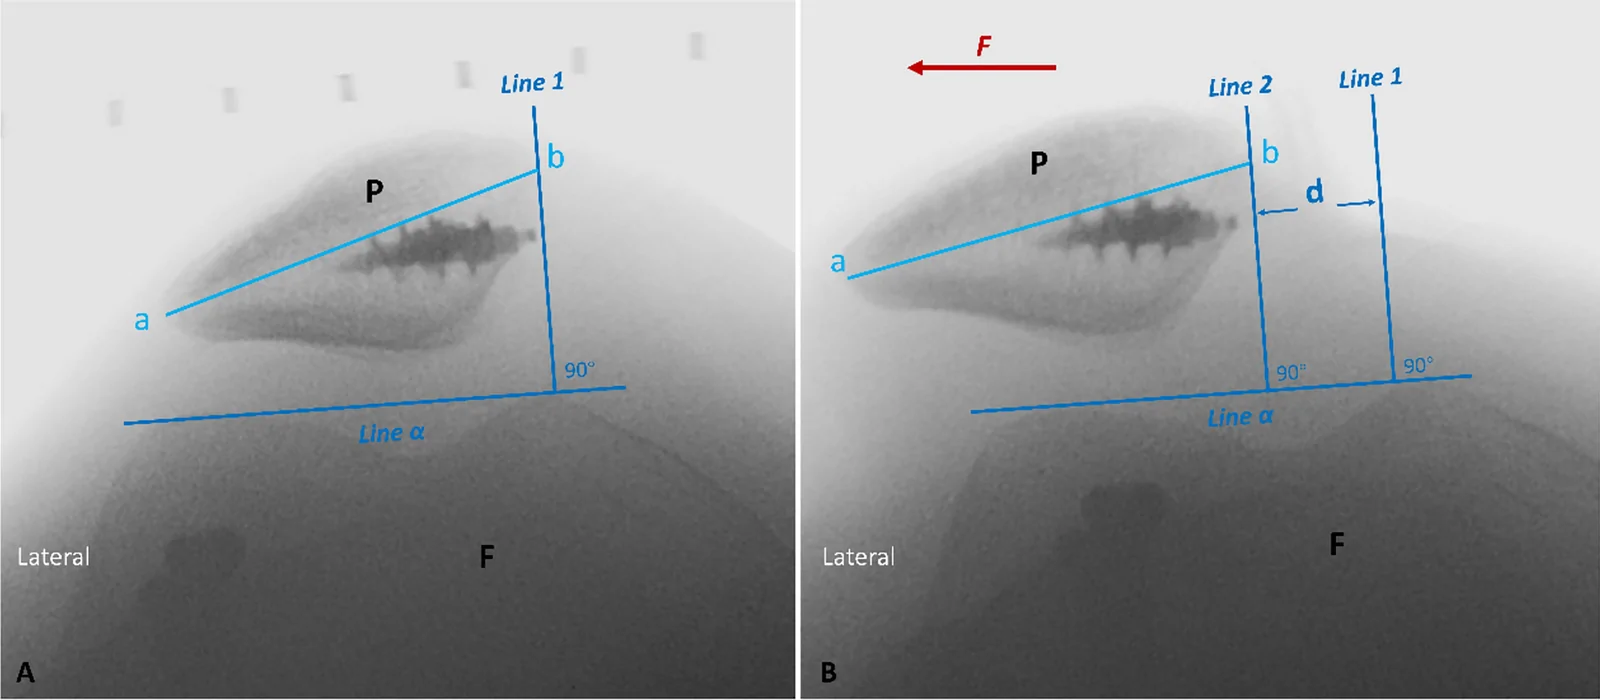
Quantitative evaluation of the patellar medial laxity index at final follow-up. (A) At 30° knee flexion in the neutral position, three key radiographic markers were established: (1) line ab representing the mediolateral patellar width, (2) line *α* drawn tangent to the femoral trochlear groove, and (3) line 1 perpendicular to *α* and intersecting the medial patellar border. (B) With 30° knee flexion under standardized lateral patellar stress (*F*), line 2 was similarly constructed perpendicular to *α* from the stressed medial patellar border. The MPFL laxity index (%) was then calculated by measuring the vertical displacement (d) between lines 1 and 2 relative to patellar width (d/ab × 100%). P, patella; F, femur
